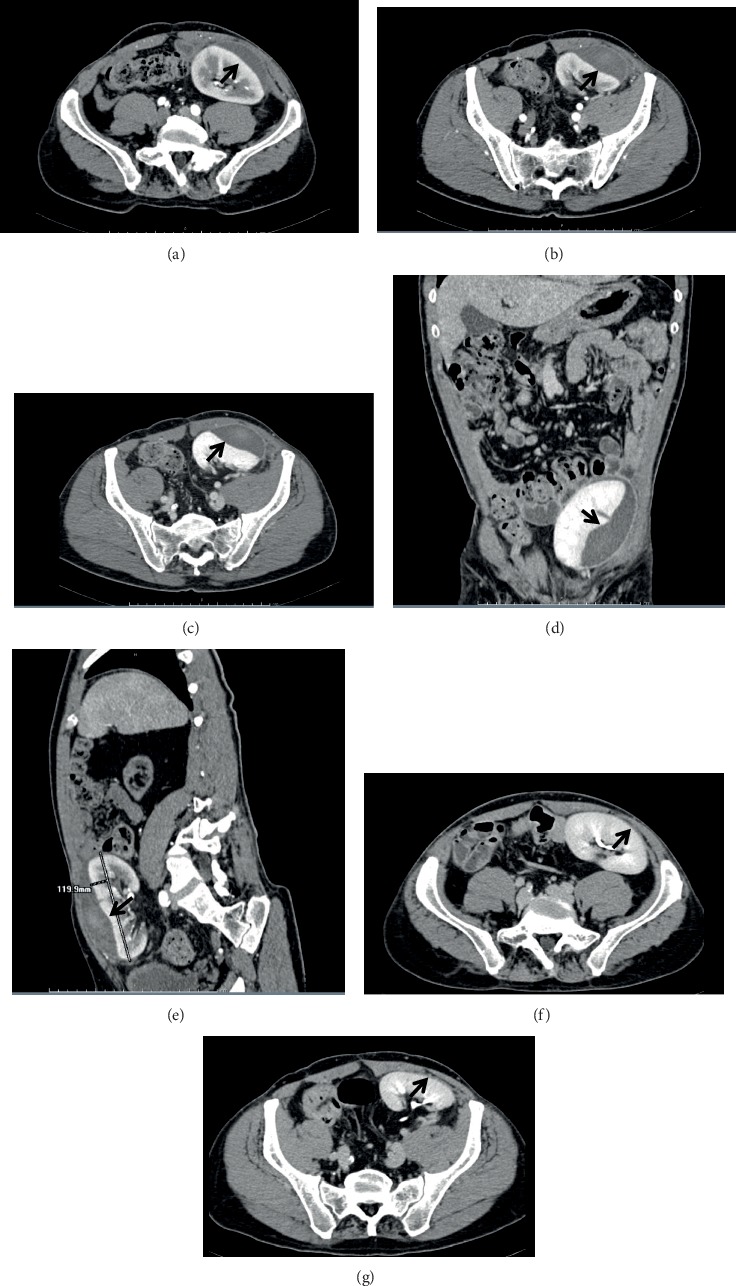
Contrast-enhanced computerized tomography (CT) scans of the transplanted kidney. (a–e) CT scan at admission showing the subcapsular hematoma (arrows). (a, b) Arterial phase. (c) Venous phase. (d) Coronal reconstruction. (e) Sagittal reconstruction. (f, g) CT scan at 4-month follow-up control showing the resolution of the subcapsular hematoma (late arterial phase). In (f, g), the arrows indicate the site of the previous hematoma.
